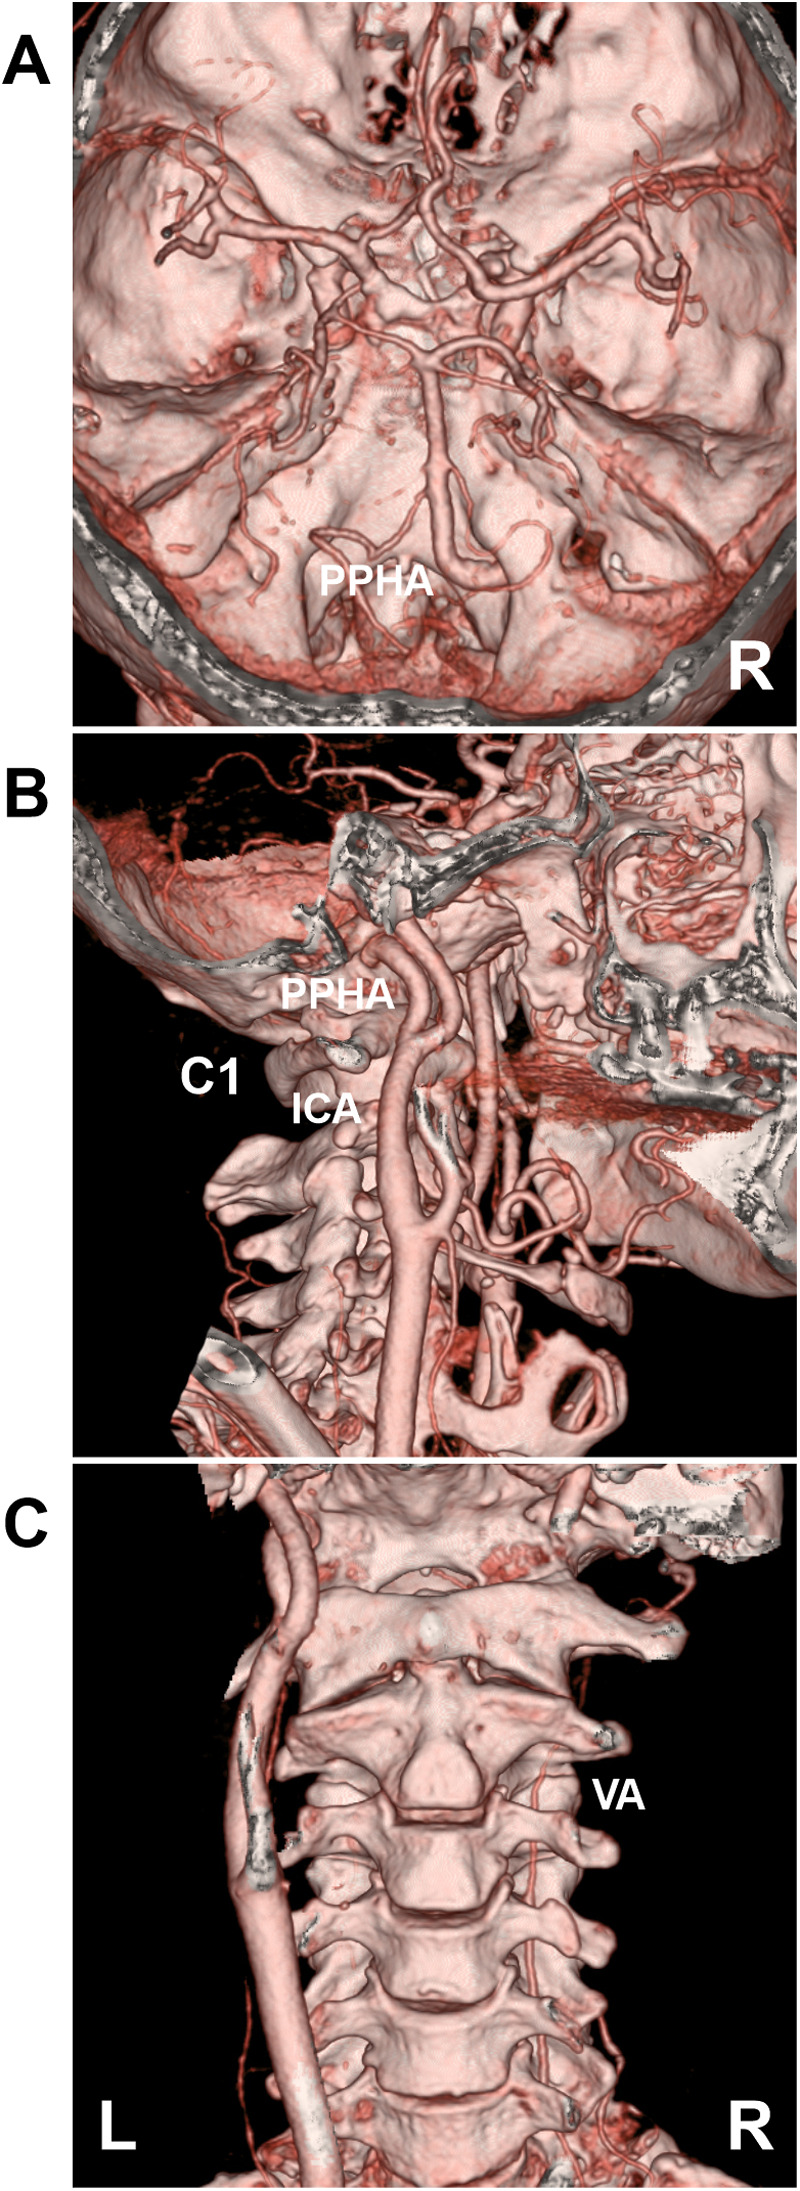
Angiographic anatomy of the PPHA on CTA. (A) CTA shows a right PPHA passing through the hypoglossal canal to replace the ipsilateral VA and joins the basilar artery. (B) CTA shows the PPHA arising from the C1 level of the ICA and ascending through the hypoglossal canal. (C) CTA shows contralateral (left) VA absence with ipsilateral (right) VA hypoplasia. C1, first cervical vertebra; CTA, computed tomography angiography; ICA, internal carotid artery; L, left; PPHA, persistent primitive hypoglossal artery; R, right; VA, vertebral artery.
